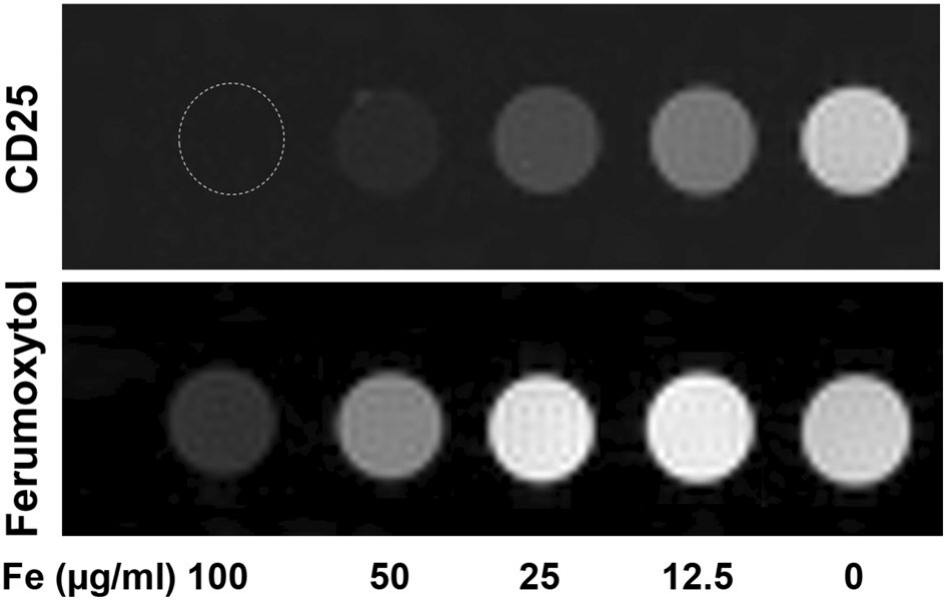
Magnetic resonance signal effects of CD25 microbeads compared to ferumoxytol. T2-weighted magnetic resonance images of agarose gels (200 µl) containing increasing contrast agent concentrations (0, 12.5, 25, 50 or 100 µg/ml of CD25 microbeads or ferumoxytol) show marked signal decrease (dark) for both contrast agents compared to controls (0 = agarose gel alone). Signal decay is more pronounced for CD25 microbeads compared to ferumoxytol. Data was acquired on a 7 T MRI with 10 TEs equally spaced from 6.4 to 64 ms.
